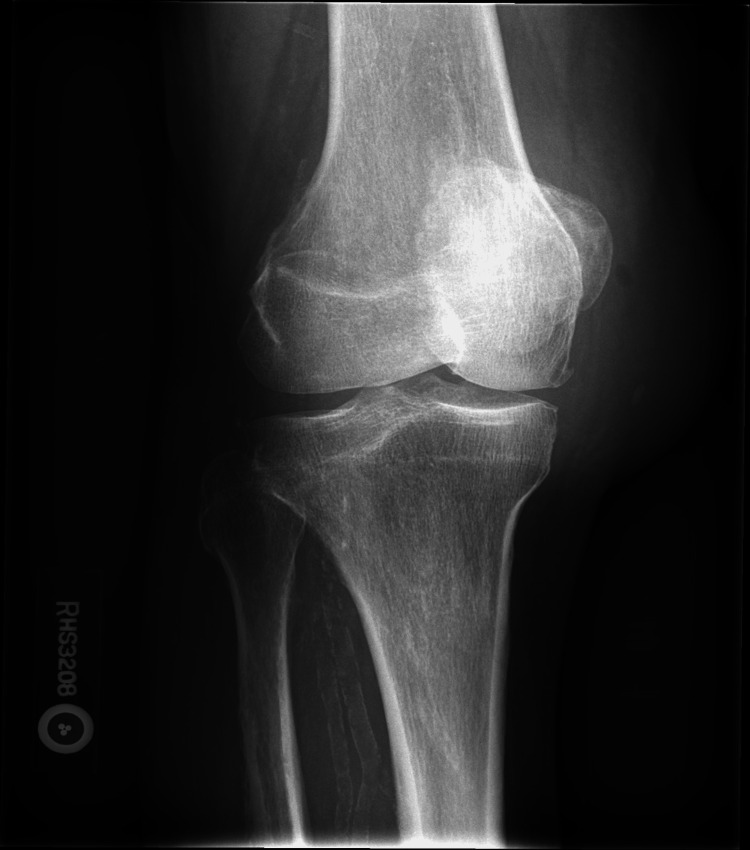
Anteroposterior view of patient’s right knee X-ray showing significant prepatellar swelling
